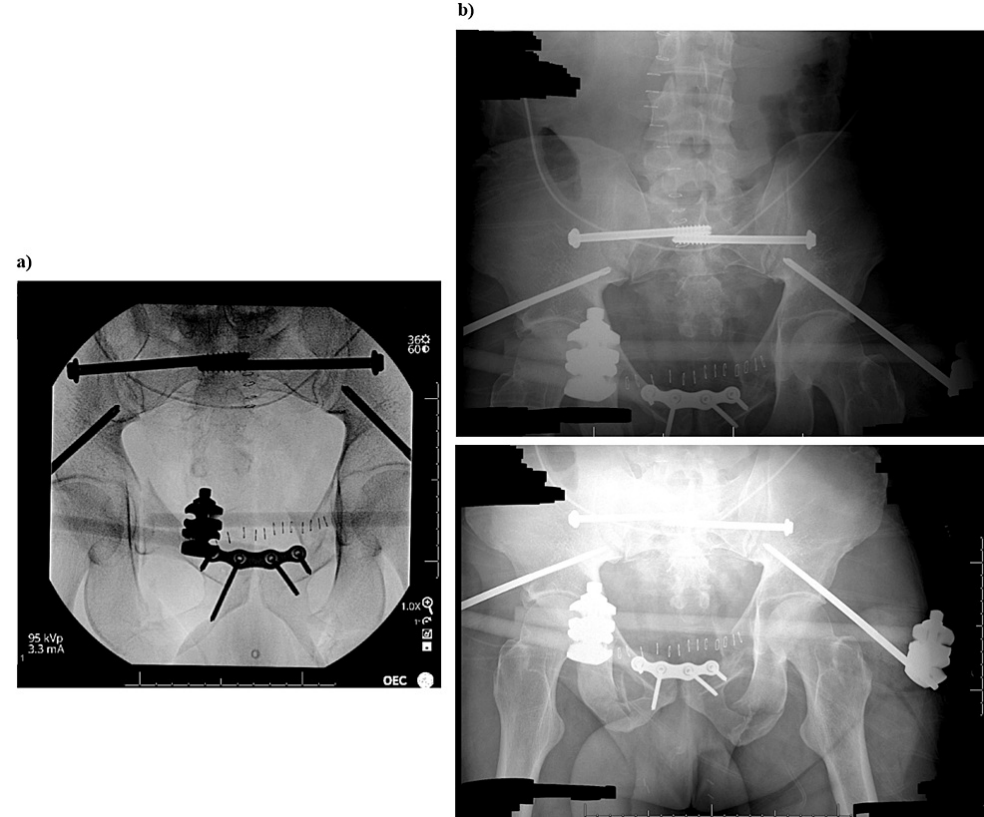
Pelvic X-rays of the external fixture after open-reduction internal fixation (ORIF). (a) Pelvic X-rays obtained using procedural fluoroscopic guidance during hardware fixation of the previously described multiple pelvic fractures. (b) Status post-ORIF of the pelvis with satisfactory anatomic alignment of the fractured fragments and no unexpected radiopaque foreign bodies seen. Overlying hardware appears intact.
